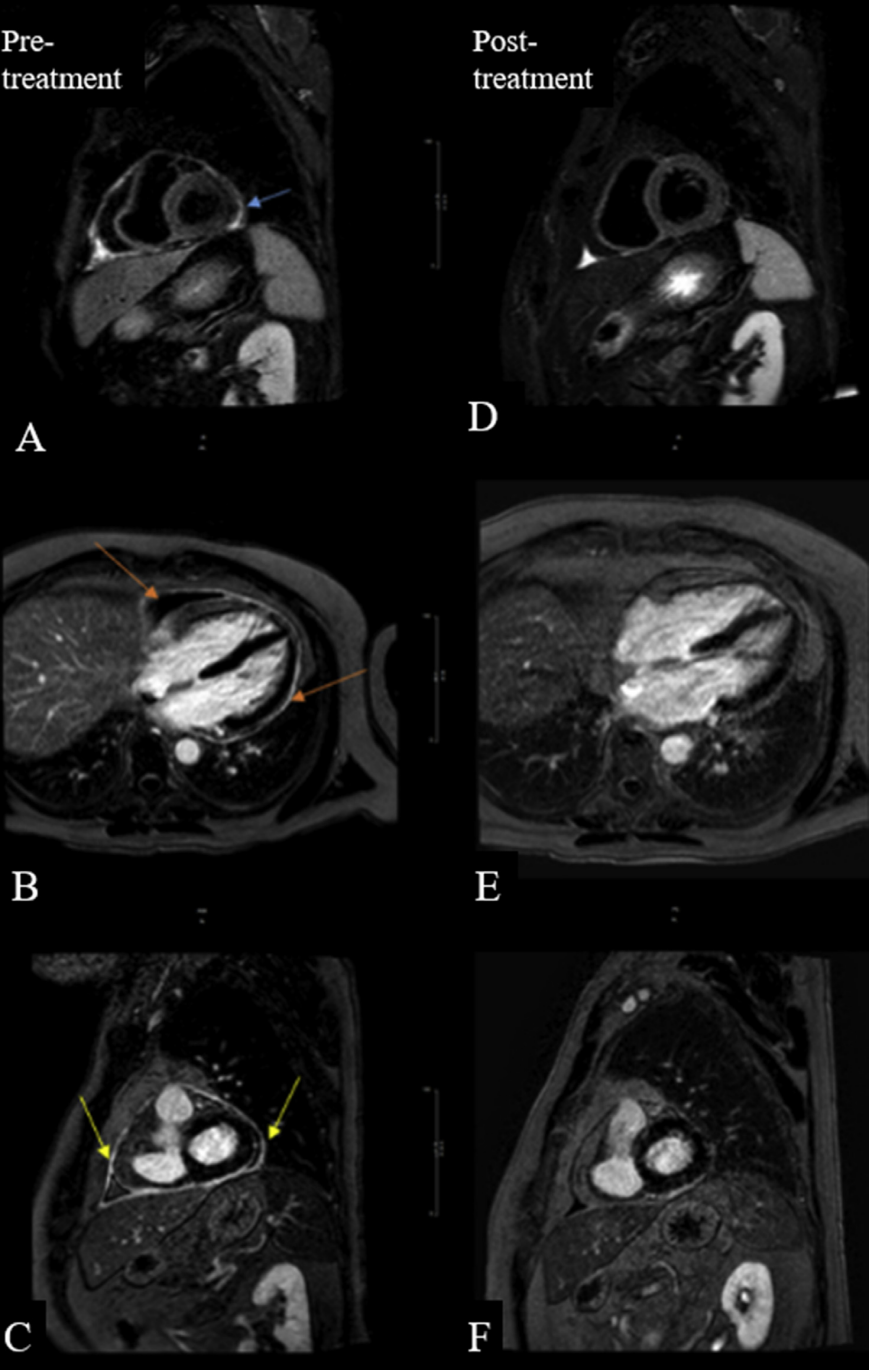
Cardiac MRI with pretreatment images showing (A) increased T2 STIR signal intensity indicating acute inflammation, small pericardial effusion, and late gadolinium enhancement in (B) four-chamber and (C) short-axis views. Cardiac MRI posttreatment images showing no more increased pericardial signal T2 STIR, indicating edema has resolved (D); resolution of late gadolinium enhancement in (E) four-chamber (F) short-axis views indicating the pericardial inflammation is resolving.
